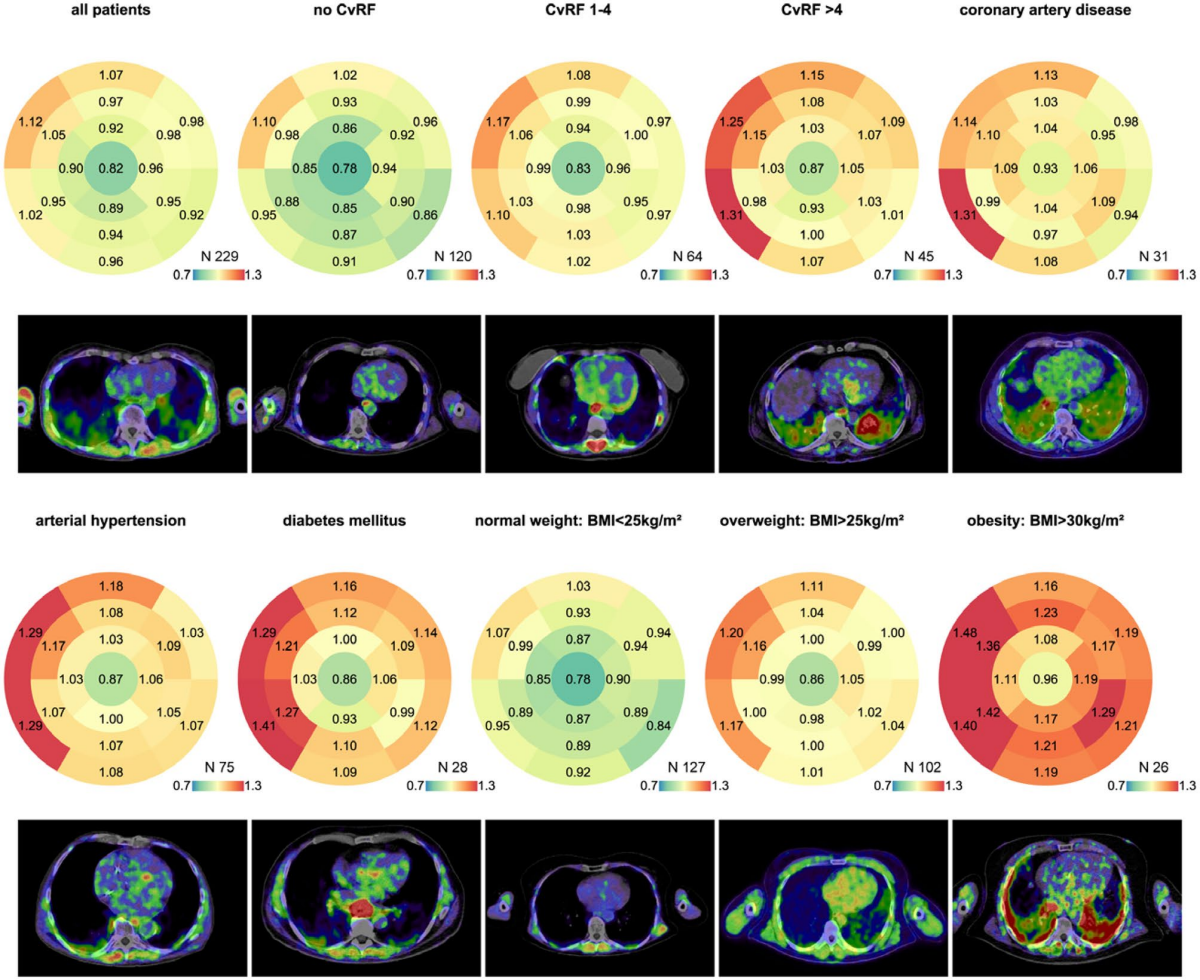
Retrospective assessment of oncology patients using 17 segment polar map analysis demonstrates progressive association of increasing cardiovascular risk factors (CvRF) with higher FAPI PET signal in the myocardium. The presence of coronary artery disease, arterial hypertension, or diabetes mellitus as well as rising body mass index (BMI) were further associated with higher FAPI signal particularly in the septal wall. Quantitative measurements are presented as median SUV, and a representative transaxial slice is presented below the polar maps. Reproduced from [26], with permission
